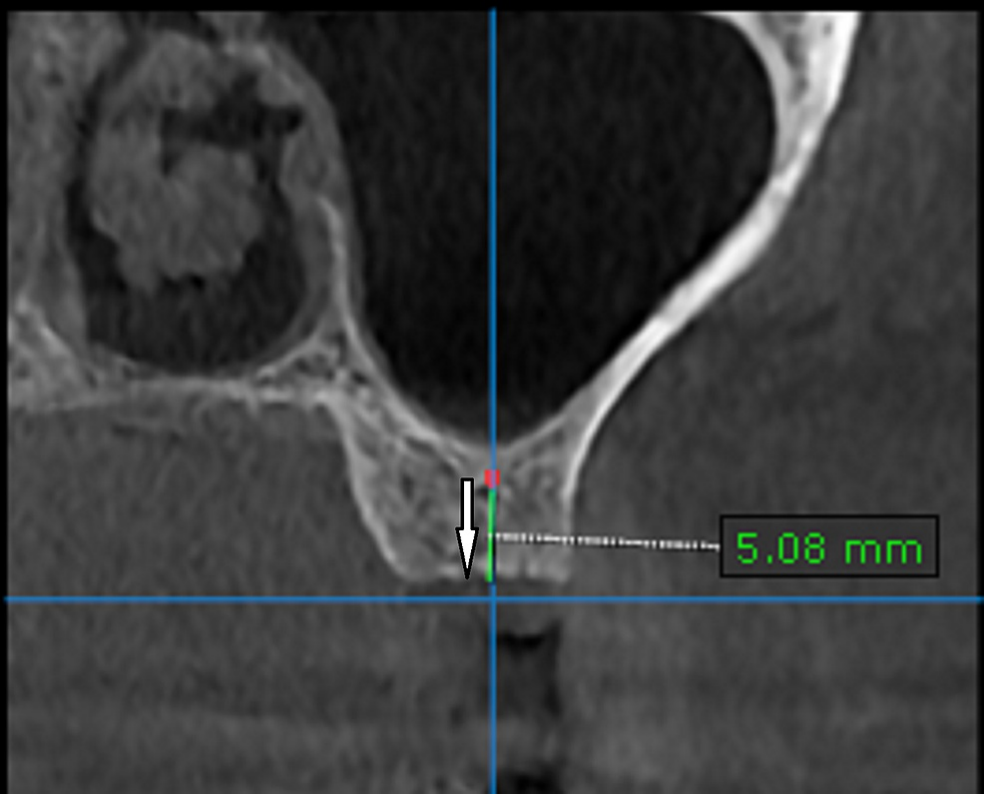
The CBCT scan of left upper first molar region taken three months after socket preservation with DFDBA. The arrow is depicting the increase in the bone volume after socket preservation. DFDBA: demineralized freeze-dried bone allograft
